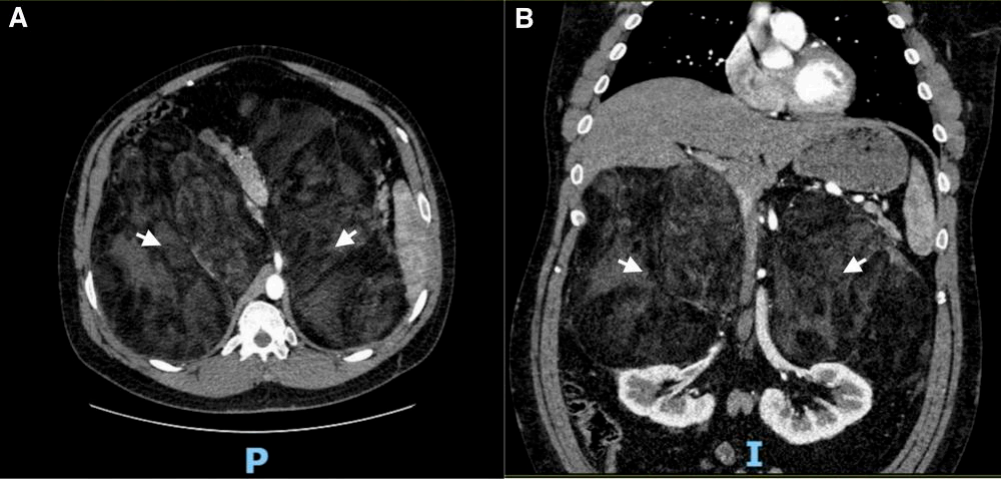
Computed tomography angiogram (CTA) abdomen with and without contrast in (A) axial view and (B) coronal view. (P) Indicating posterior side and (I) indicating inferior side. White arrows indicate adrenal masses. The right adrenal lesion measures 24.3 × 17.4 centimeters (cm). The left adrenal lesion measures 27.4 × 13.4 cm. Bilateral renal arteries effaced inferiorly secondary to mass effect from the large bilateral macroscopic fat-containing adrenal masses. The inferior vena cava is compressed by the large right retroperitoneal mass without evidence of occlusion or thrombosis. The kidneys are displaced inferiorly secondary to the adrenal masses. The unenhanced CT attenuations of the masses were −41.50 Hounsfield units (HU)for the right mass and −55.64 HU for the left mass.
